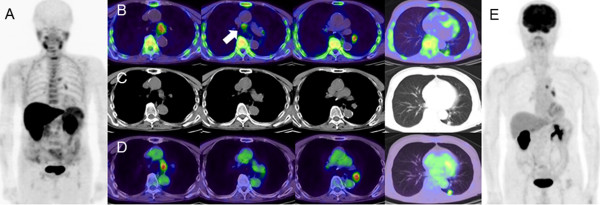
4DST MIP (A), 4DST axial (B), CT (C), FDG axial (D), and FDG MIP images (E). PET images (axial and maximum-intensity-projection) with 4DST and FDG for lymph node lesions and the primary lung cancer in patient No. 23. The background uptake is clearly lower in 4DST than FDG. 4DST and FDG show intense uptake at the left lower paratracheal lymph node (#4 L) and the left hilar lymph node. 4DST also shows uptake at the lower paratracheal lymph node (arrow), not clearly identified on FDG.
